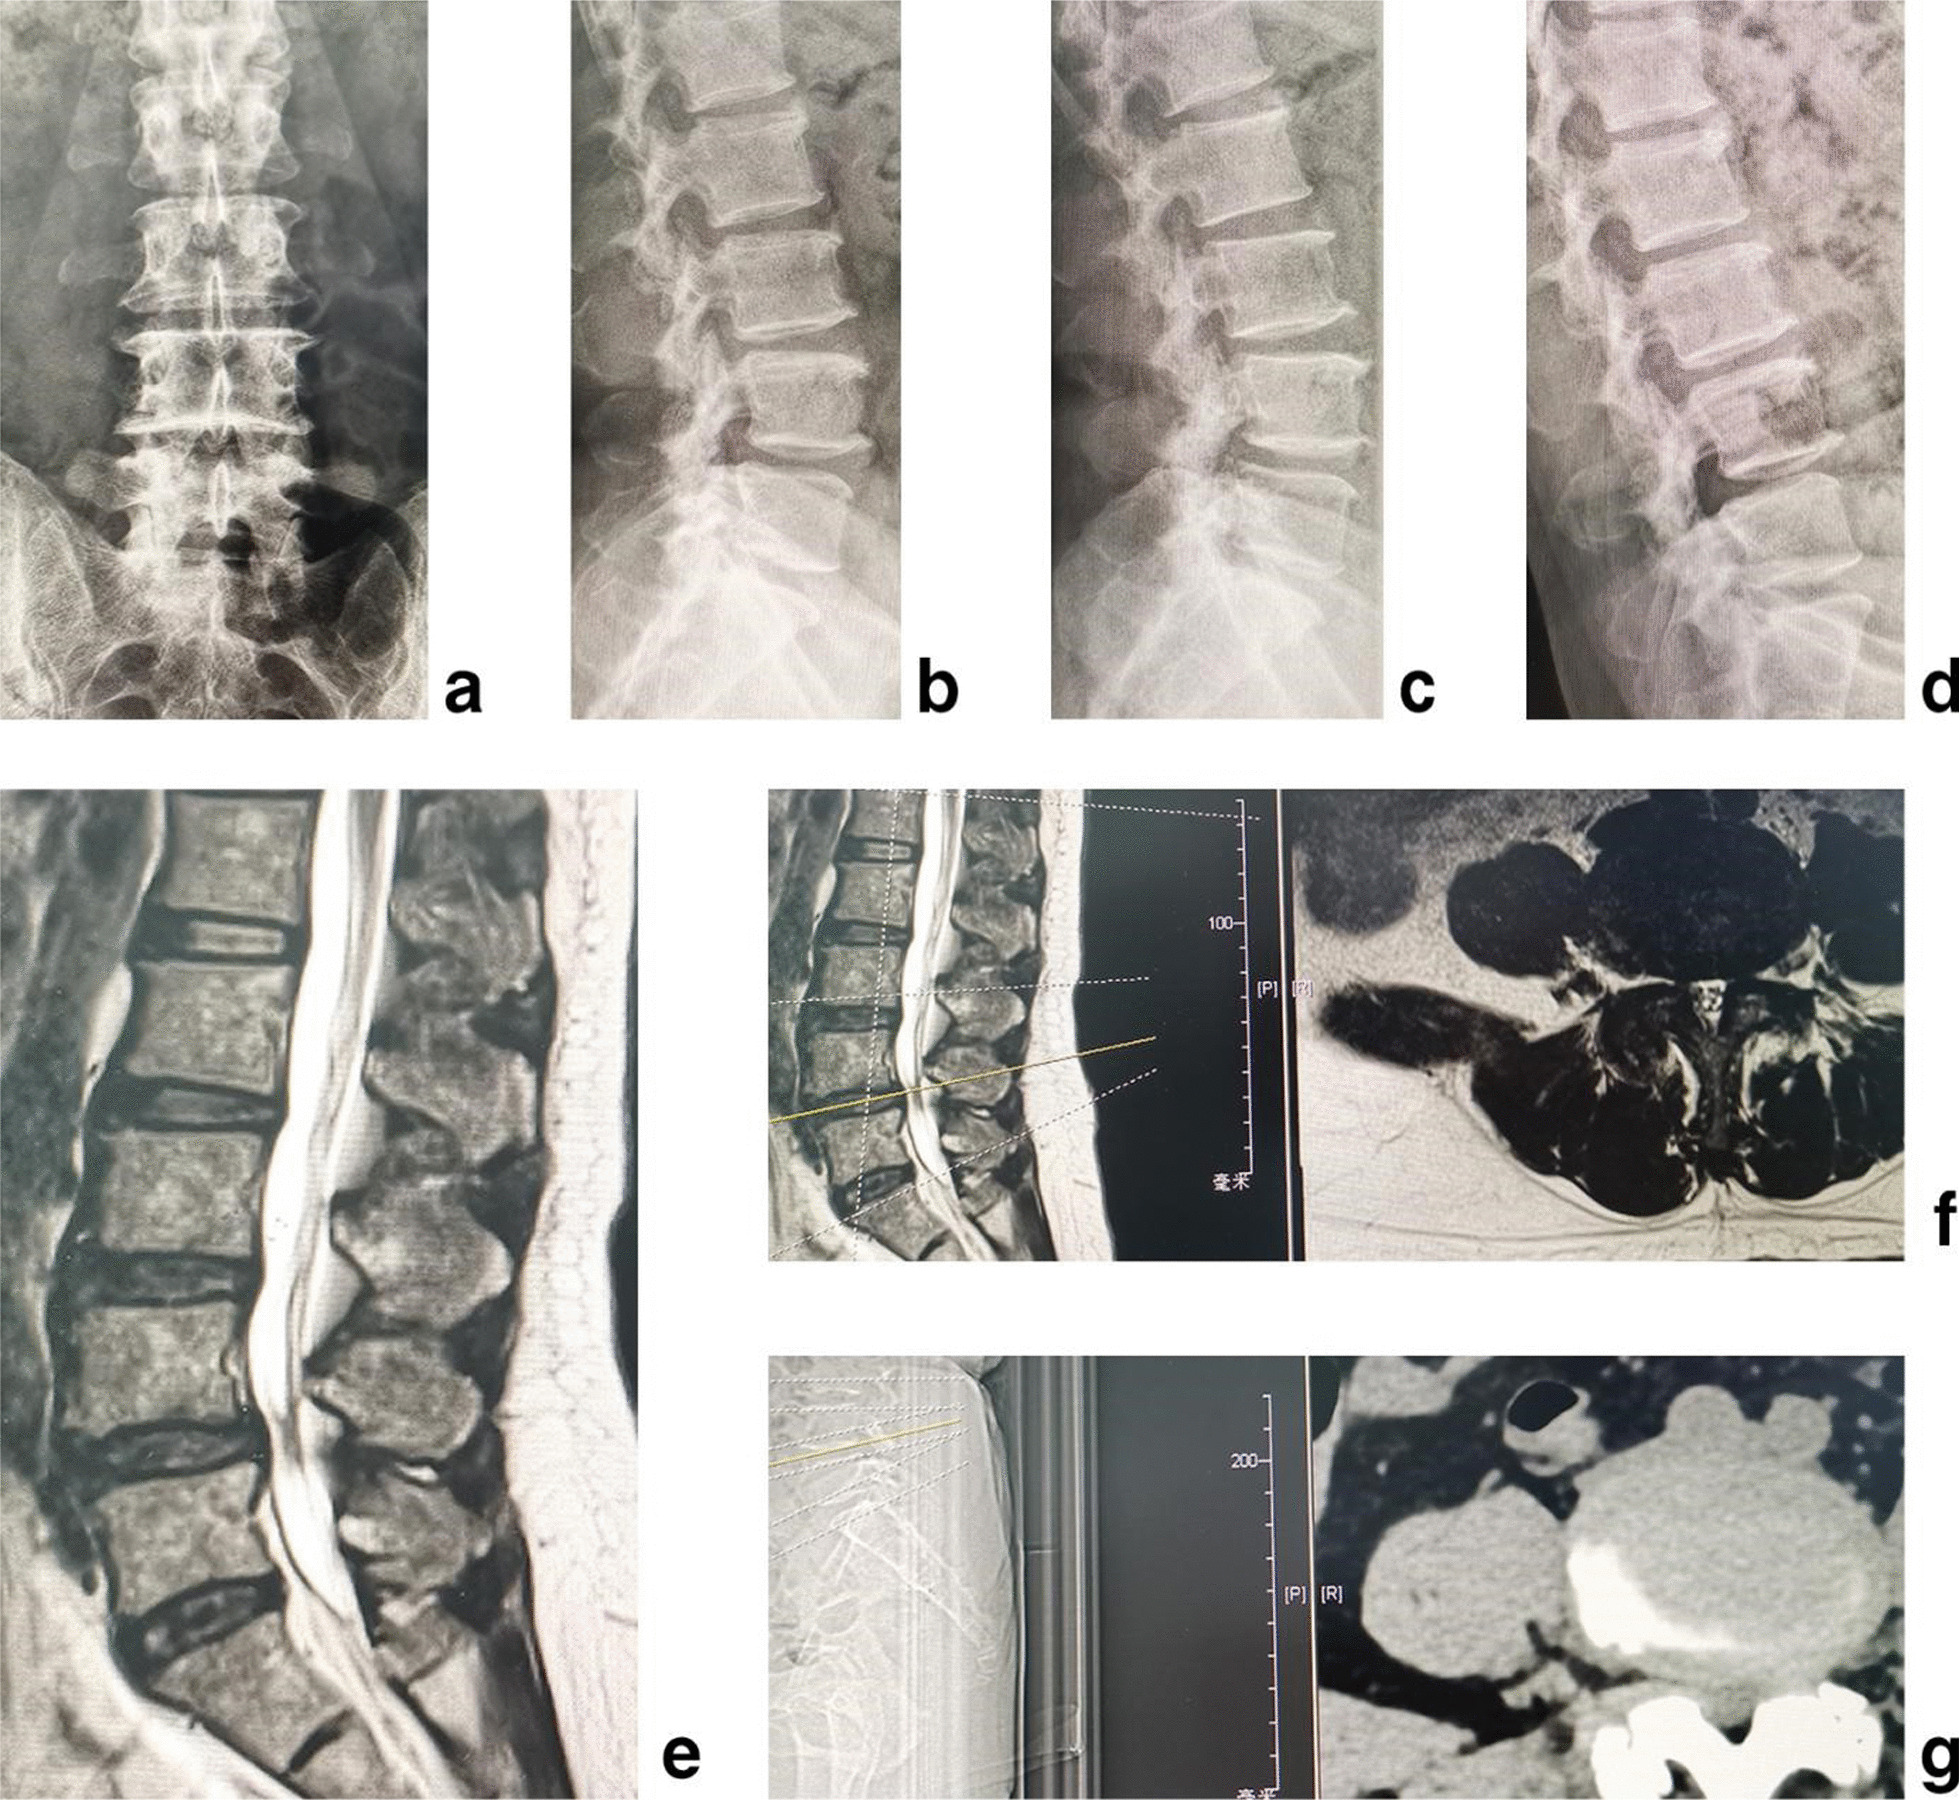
The female patient of 58 years old had the pain of right lateral buttock, posterolateral thigh, lateral calf and dorsal foot. a Posteroanterior X-ray, b lateral X-ray, c hyperextension lateral X-ray, d hyperflexion lateral X-ray, e sagittal MR image, f axial MRI and g axial CT showed multi-level lumbar degeneration of L3/4, L4/5, L5/S1 with L4/5 instability. It was diagnosed with multi-level LDDs and L4/5 instability
